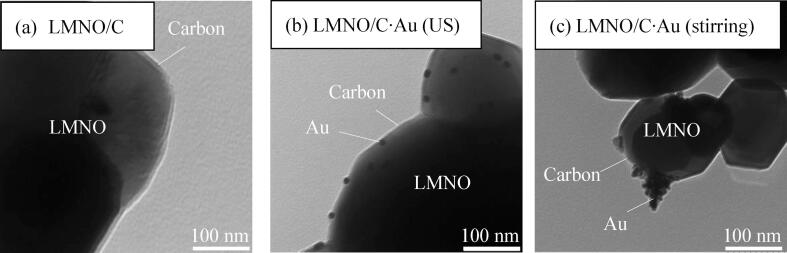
TEM images of (a) LMNO/C (C: 1.30 wt%), (b) LMNO/C·Au prepared using ultrasound (C: 1.30 wt%, Au: 0.11 wt%), and (c) LMNO/C·Au prepared using stirring (C: 0.91 wt%, Au: 0.78 wt%).
